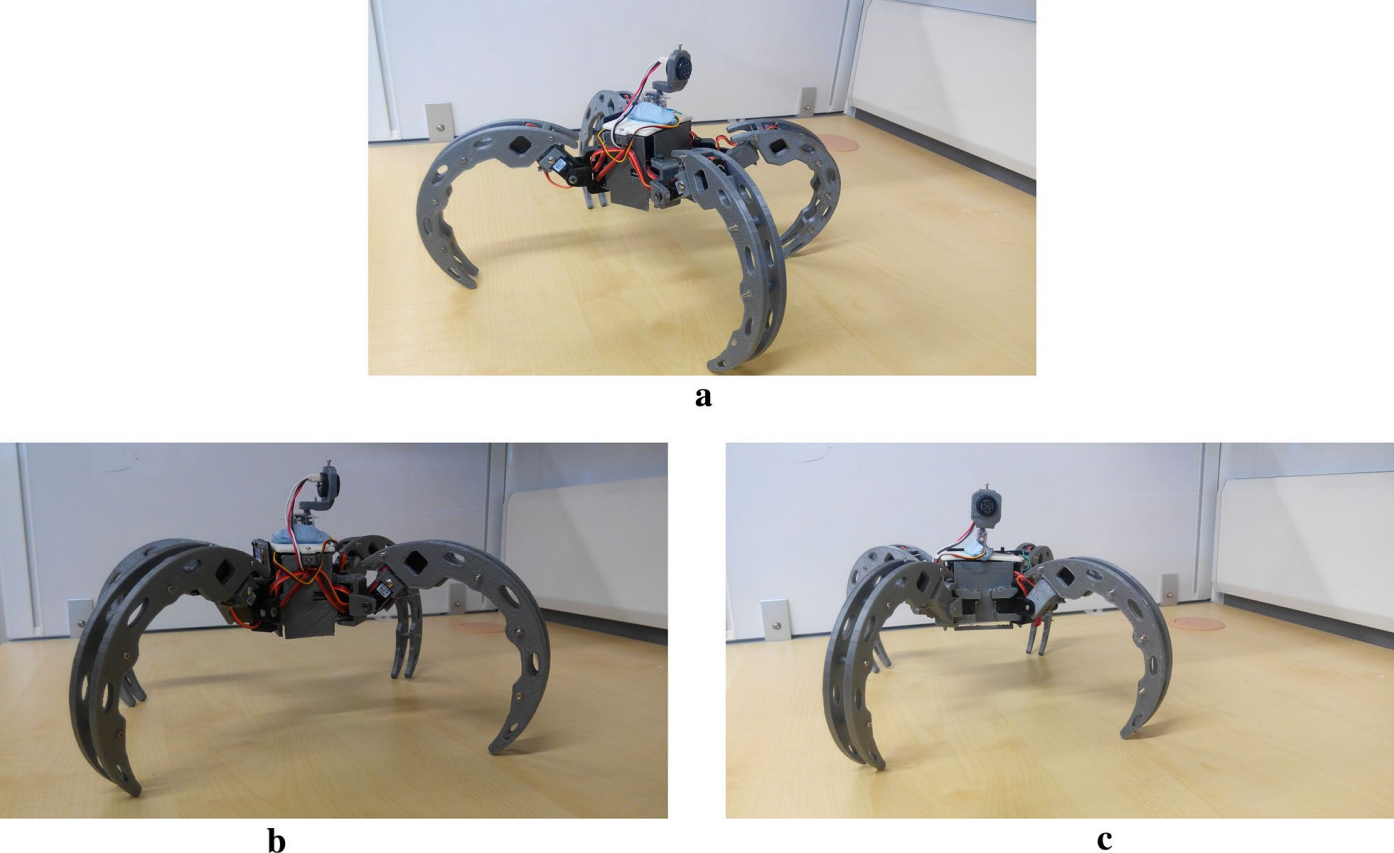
Scorpio with customized ultrasonic sensor. a Perspective view, b side view, c front view
